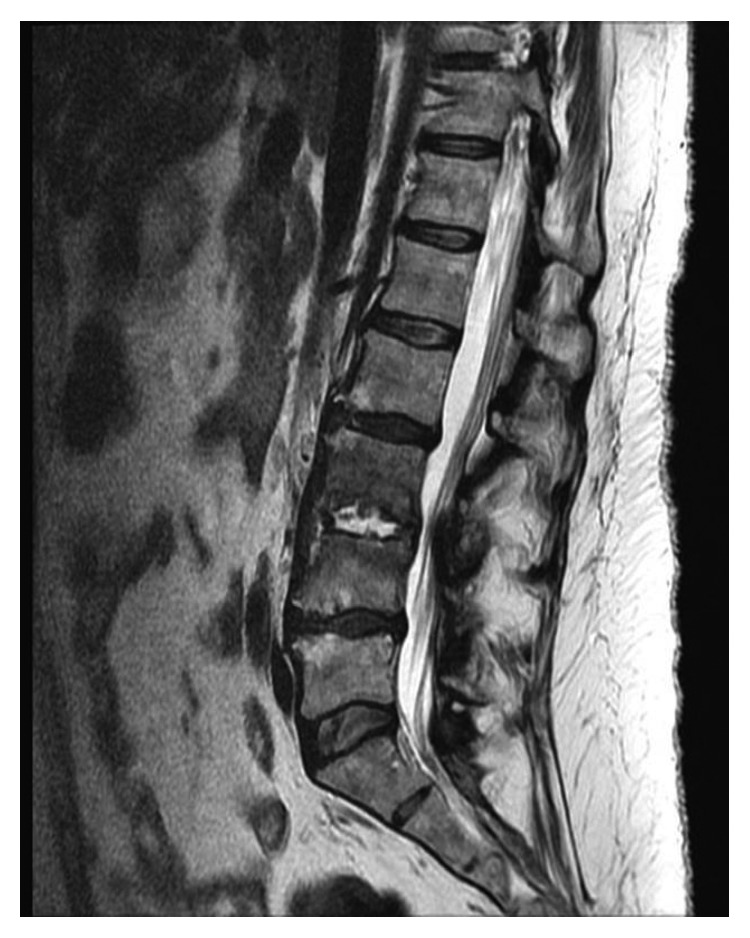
MRI thoracic spine: lateral view reveals discitis osteomyelitis at T8-T9 with an associated 1.6 × 0.8 × 1.5 cm mature rim-enhancing right anterior paravertebal abscess.
